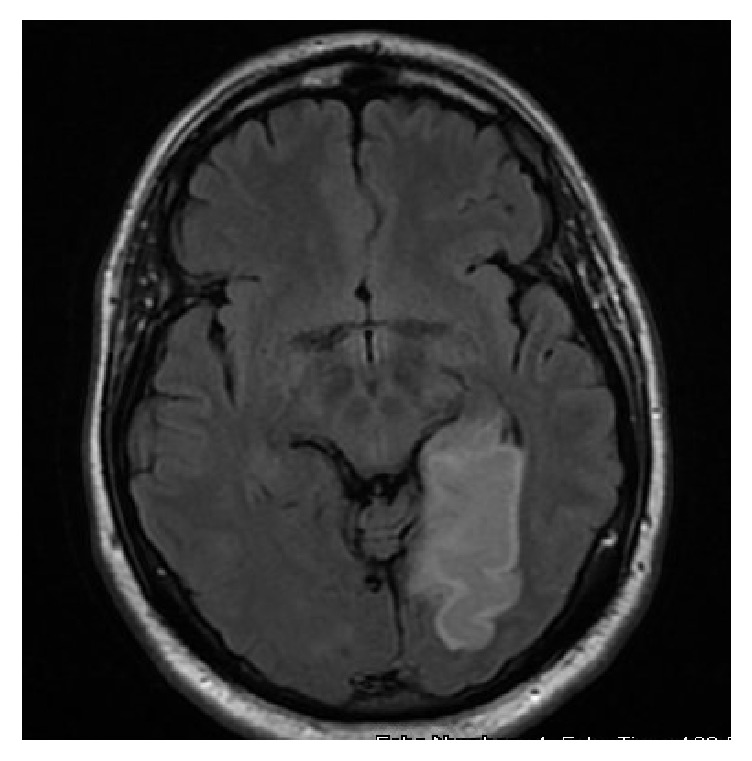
Brain MRI axial T2 FLAIR image showing left PCA infarction.
